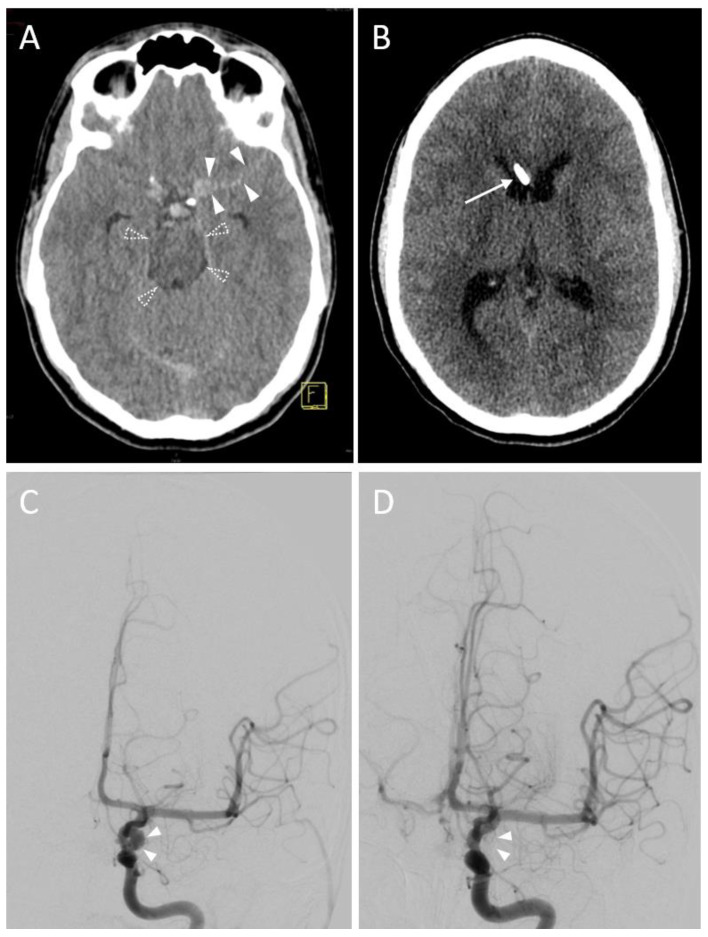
A 16-year-old male with a subarachnoid hemorrhage from an aneurysm of the left internal carotid artery. (A) Planning a CT scan revealed a subarachnoid hemorrhage (Fisher Grade IV). Blood collections are shown in the perimesencephalic cistern (transparent arrowheads) and the lateral fissure (opaque arrowheads). Due to beginning hydrocephalus, indication for EVD placement was given. Yellow graphic: orientation cube (f: frontal). (B) Control CT scan. Successful EVD placement in the right frontal horn with tip next to the septum pellucidum (Kakarla I). (C) Digital Subtraction Angiography (DSA) before endovascular treatment. Source of bleeding is a broad-based saccular aneurysm at the outlet of the left posterior communicating artery with a maximum diameter of 6.5 × 5.0 mm (arrowheads). (D) DSA control scan after coil placement depicts complete elimination of the aneurysm sac.
